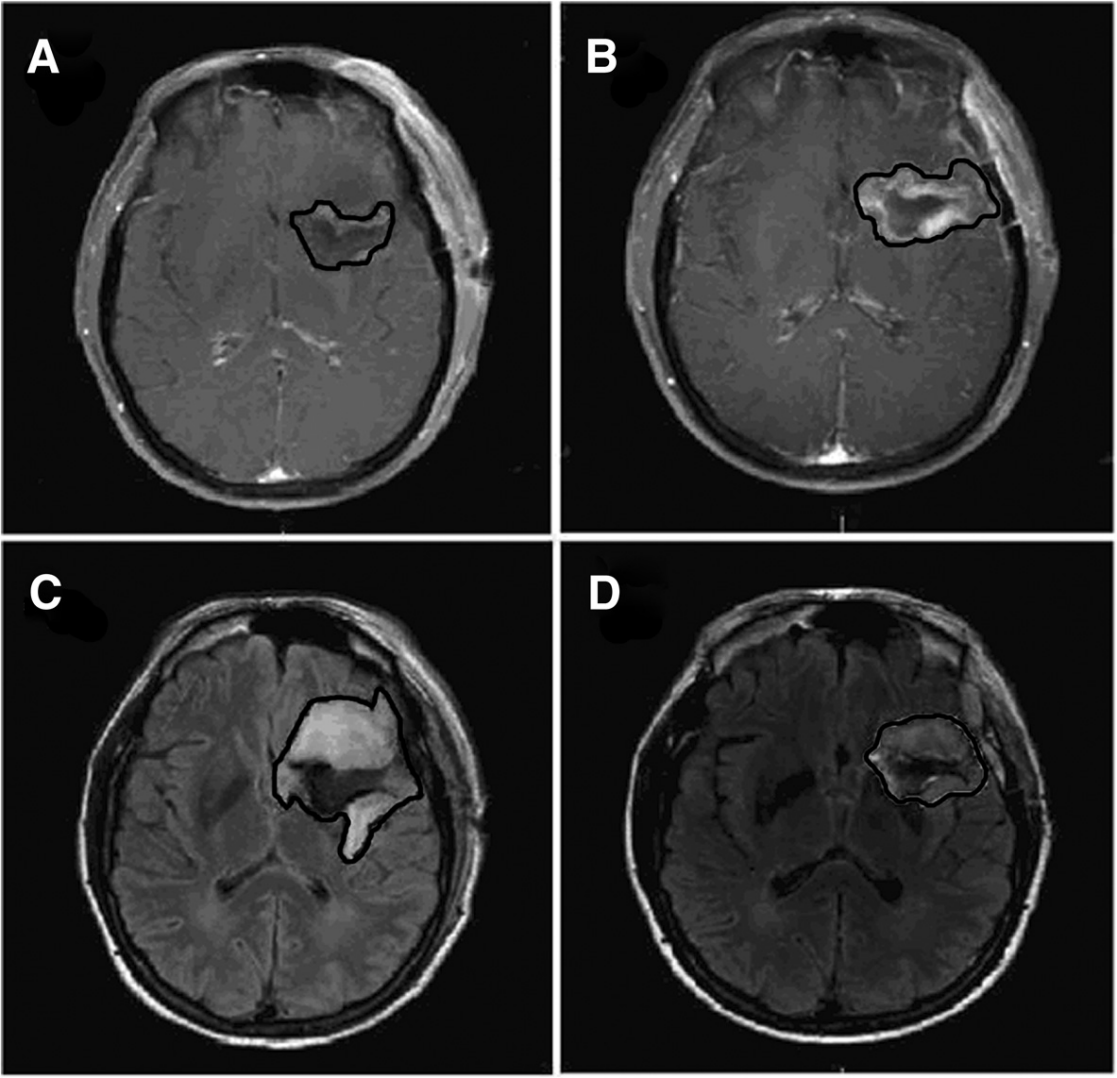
Changes of GTV on T2/FLAIR and contrast-enhanced MRI: A. GTV2 on post-operative T1 contrast-enhanced MRI. B. GTV2 on delayed T1 contrast-enhanced MRI, C. GTV1 on post-operative T2/FLAIR MRI. D. GTV1 on delayed T2/FLAIR MRI.
